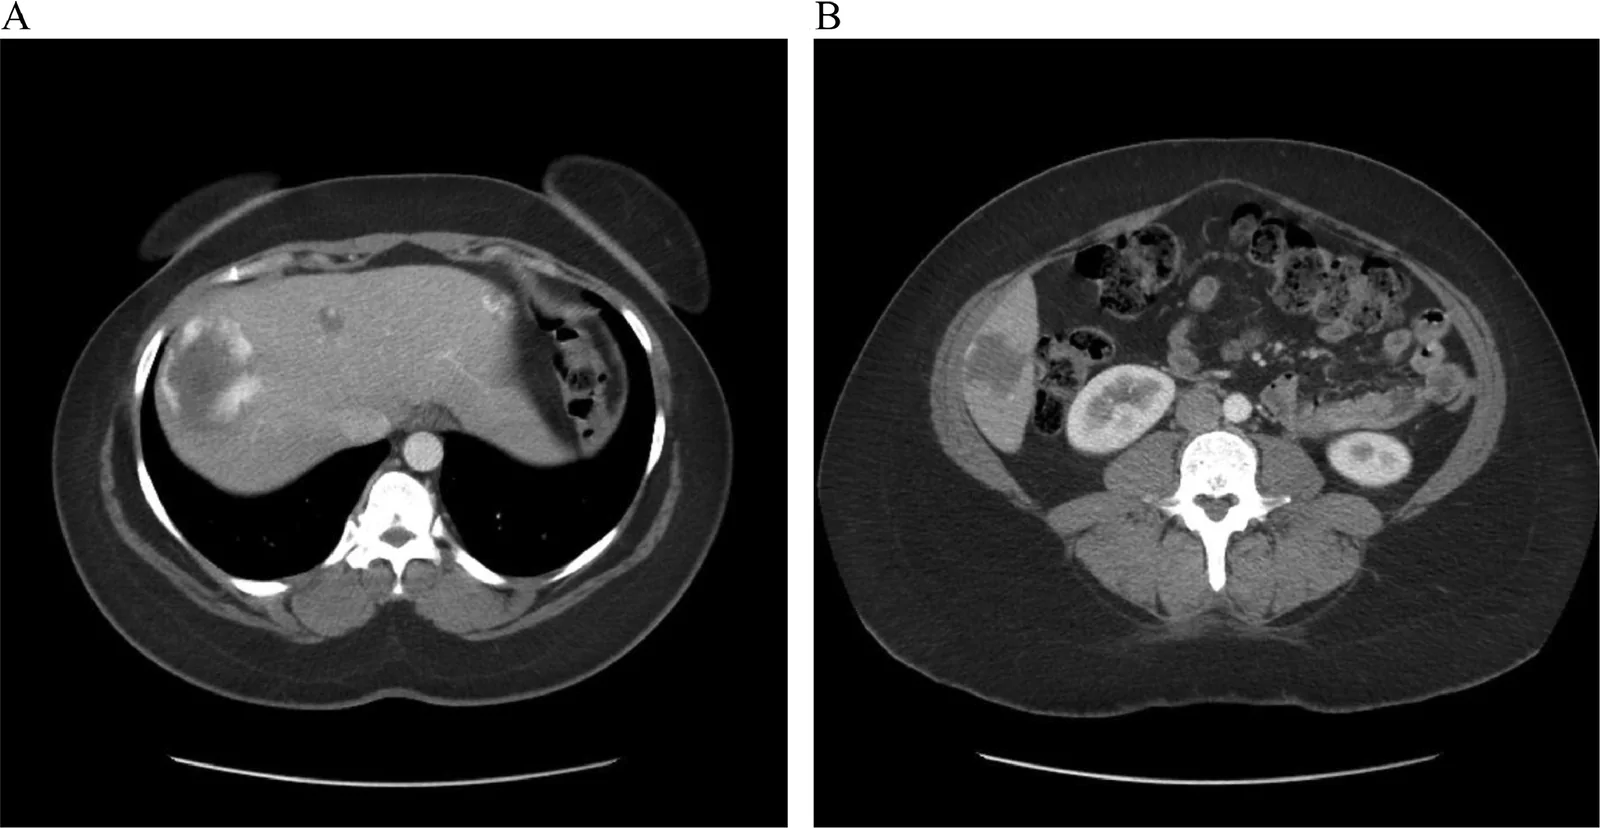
Axial CT findings of multifocal hepatic hemangiomas. (A) Contrast-enhanced CT demonstrates a subdiaphragmatic hypodense lesion with peripheral contrast enhancement in segment 7/8 measuring 5.1 × 5.9 cm on the axial view (craniocaudal diameter 6.4 cm, measured on the sagittal view; not shown). (B) Contrast-enhanced CT demonstrates an inferior hypodense lesion with peripheral contrast enhancement in segment 6 measuring 3.0 × 3.5 cm on the axial view (craniocaudal diameter 3.4 cm, measured on the sagittal view; not shown).
